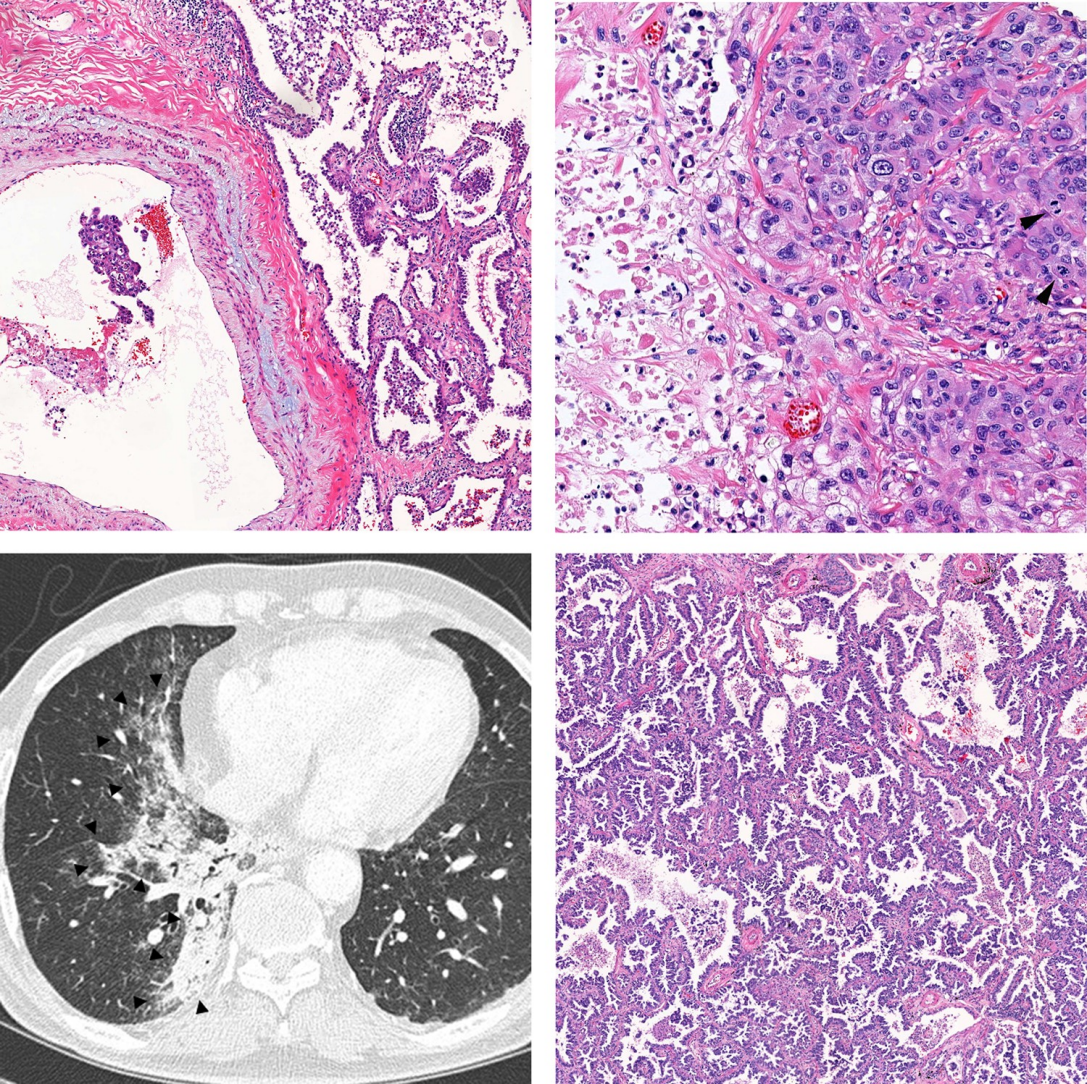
Representative cases with ctDNA shedding in plasma. A-B, A solid predominant adenocarcinoma with EGFR L858R mutation in both tissue and plasma (case no. 35). The tumor showed vascular invasion (A, ×40), necrosis (B; left side of the picture, ×200), cytological atypia, and frequent mitosis (B, arrow heads). C-D, A pneumonic-type adenocarcinoma with KRAS G12V mutation in both tissue and plasma (case no. 13). Large tumor in the right lower lobe on chest CT image is delineated by arrow heads (C). The tumor showed adenocarcinoma with mixed papillary and lepidic pattern (D, ×40).
